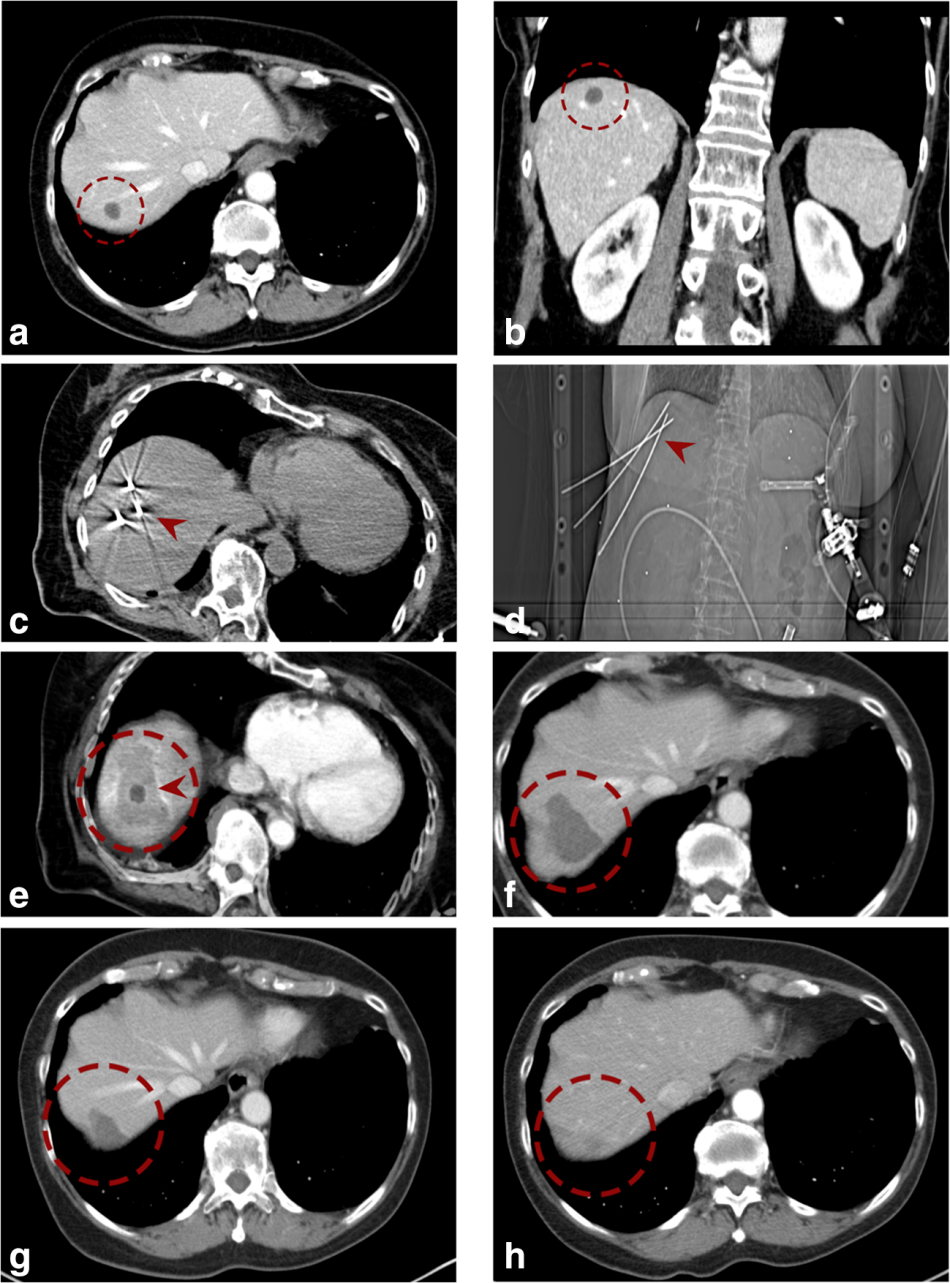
Case of a 70-year-old female with a 1.5-cm colorectal cancer liver metastasis in segment VII in the hepatic dome. a, b Portal venous phase initial CT scans with a hypo-enhancing nodule in segment VII (*red dashed circle*). c, d Axial native control CT and scout (d) with 3 coaxial needles in place (*red arrowhead*). e Fused CT image of the contrast-enhanced planning and final control CTs showing a complete coverage of the tumor (*dark central nodule*) by the coagulation zone (*red arrowhead*). f–h The *red dashed circle* is marking the progressively shrinking coagulation zone at 3 months (f), 24 months (g), and 48 months (h) after SRFA
